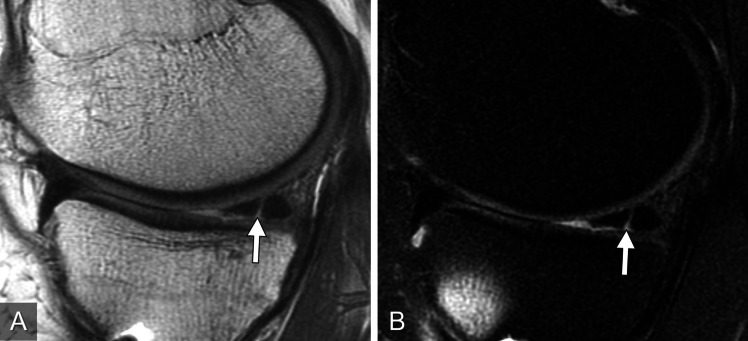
Recurrent tear of the medial meniscus in a 30-year-old woman with history of ACL reconstruction who underwent repair of a bucket-handle tear of the medial meniscus. Sagittal proton-density-weighted MRI (A) and sagittal T2-weighted fat-suppressed MRI (B) obtained one year after meniscus repair demonstrate linear fluid-like signal (arrows) surfacing at the medial meniscus posterior horn indicative of a tear, which was confirmed arthroscopically and treated with partial meniscectomy
